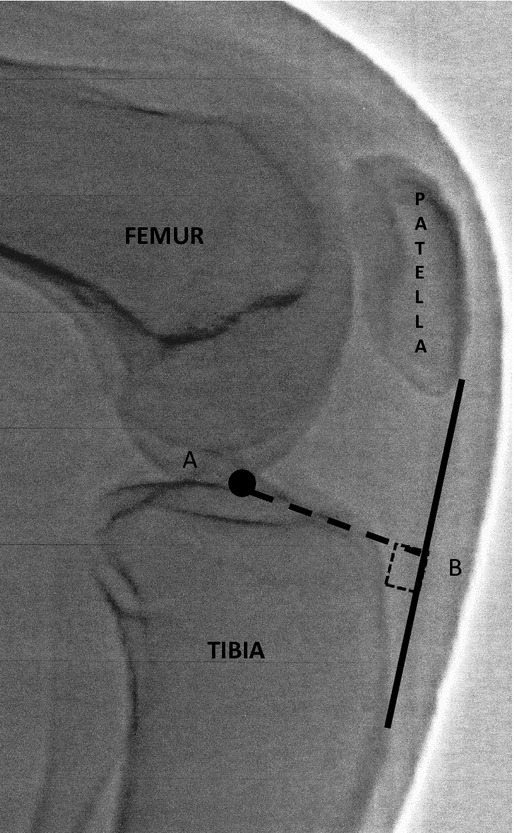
Typical sagittal plane image taken by Dual Energy X-ray Absorptiometry (DEXA) with the knee at 90^o^ flexion for measurement of patella tendon moment arm. The moment arm is the line A–B (i.e., the perpendicular distance from the joint center of rotation to the line of force application).
